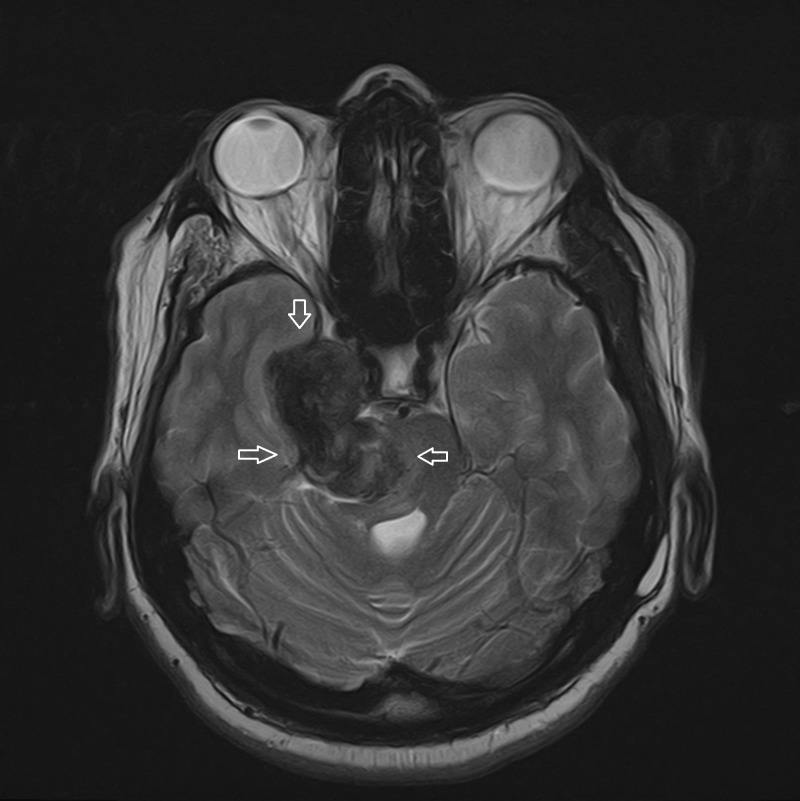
MRI T2 axial brain Significantly hypointense lesion appears to have calcified/hemorrhagic/melanotic internal contents
